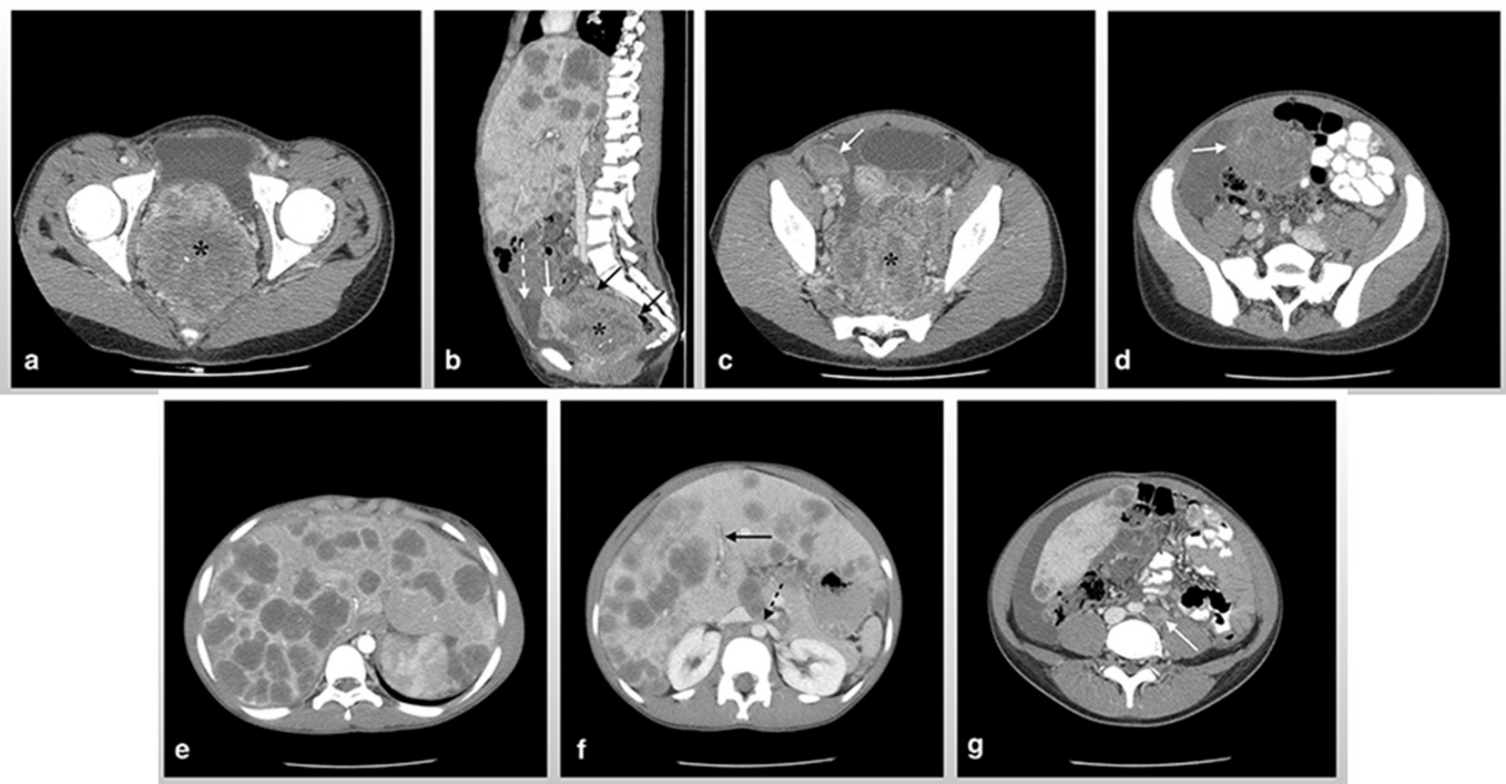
Axial CT scan image shows a large ill-defined heterogeneous solid enhancing mass (asterisk) in the pouch of Douglas within the central pelvis which demonstrates low attenuating central areas along with a few calcific foci (a). Sagittal CT scan image demonstrates mass effect on the uterus (white arrow) anteriorly without a plane of separation. The sigmoid rectum (black arrows) was collapsed and draped over the superior extent of the mass. It was also inseparable (b). Peritoneal deposits (white arrow) are seen in the right adnexa (c) and within the mesentery (d). Axial CT scan images show innumerable, predominantly rounded low attenuation (35.0 HU) masses (e), the intrahepatic portion of the inferior vena cava, hepatic veins (black arrow) and left renal vein (dotted black arrow) compressed (f) and para-aortic lymph nodes (white arrow) (g).
